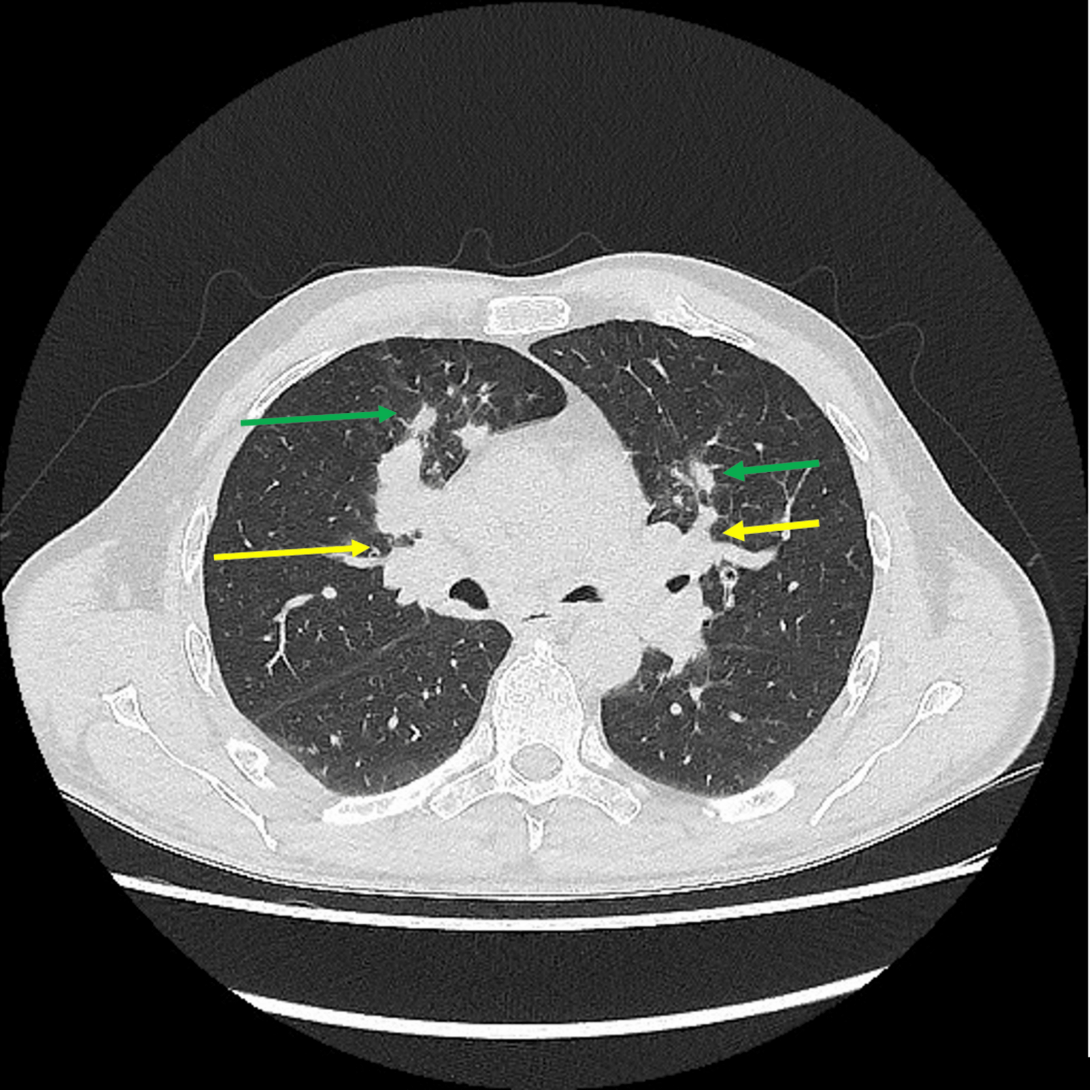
Axial high-resolution CT shows bilateral peribronchovascular nodules (green arrows) and symmetrical bilateral hilar and mediastinal lymphadenopathy (yellow arrows). These imaging findings are consistent with pulmonary involvement in granulomatosis with polyangiitis.
